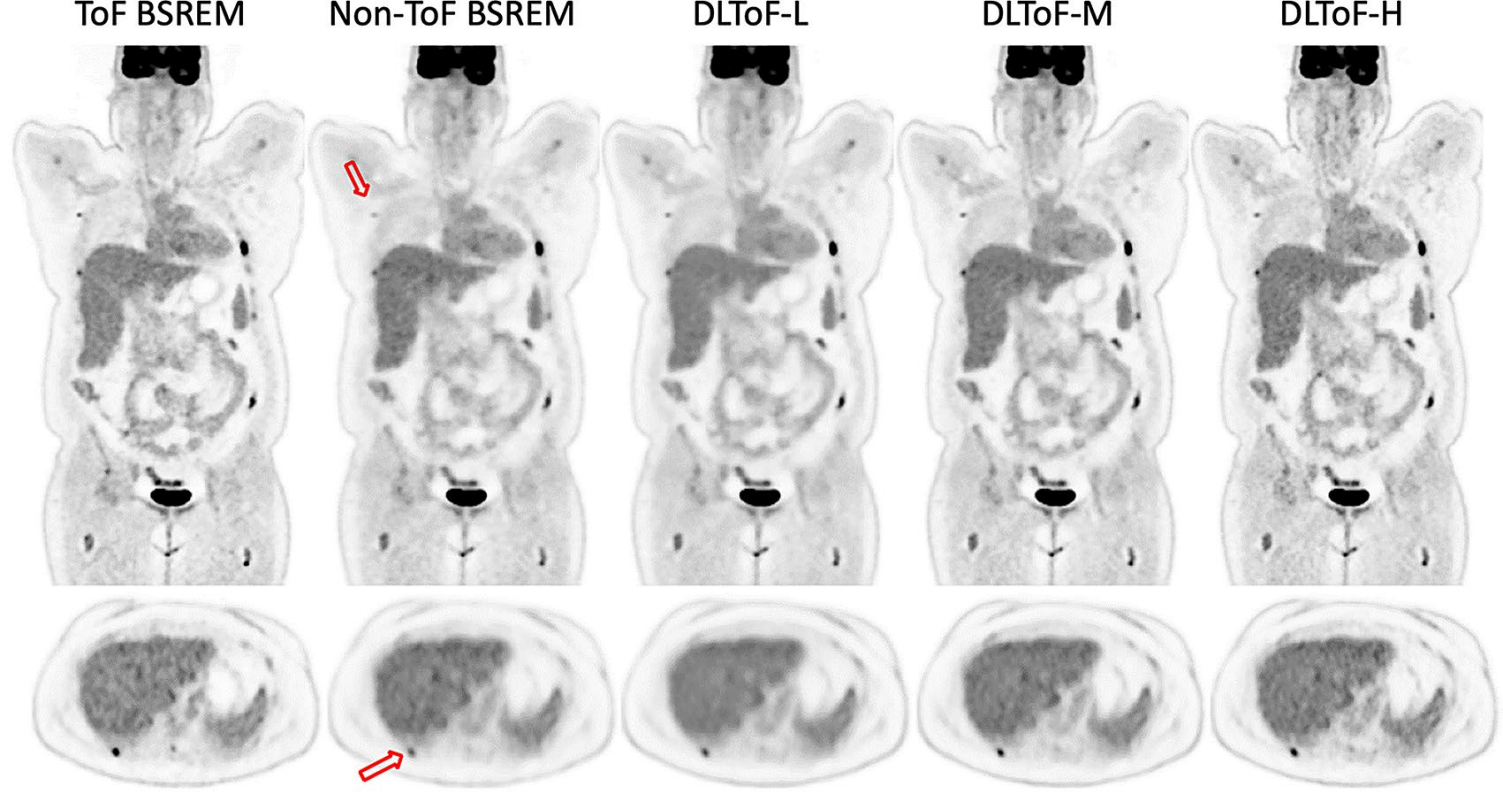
DL-ToF enhancement of an ^18^F-FDG test subject with a BMI of 34.0 kg/m^2^ with an injected activity of 521.3 MBq of scanned on DMI PET/CT scanner. Arrows point to lesions with lower conspicuity in non-ToF BSREM. Display window: 0–5 SUV
